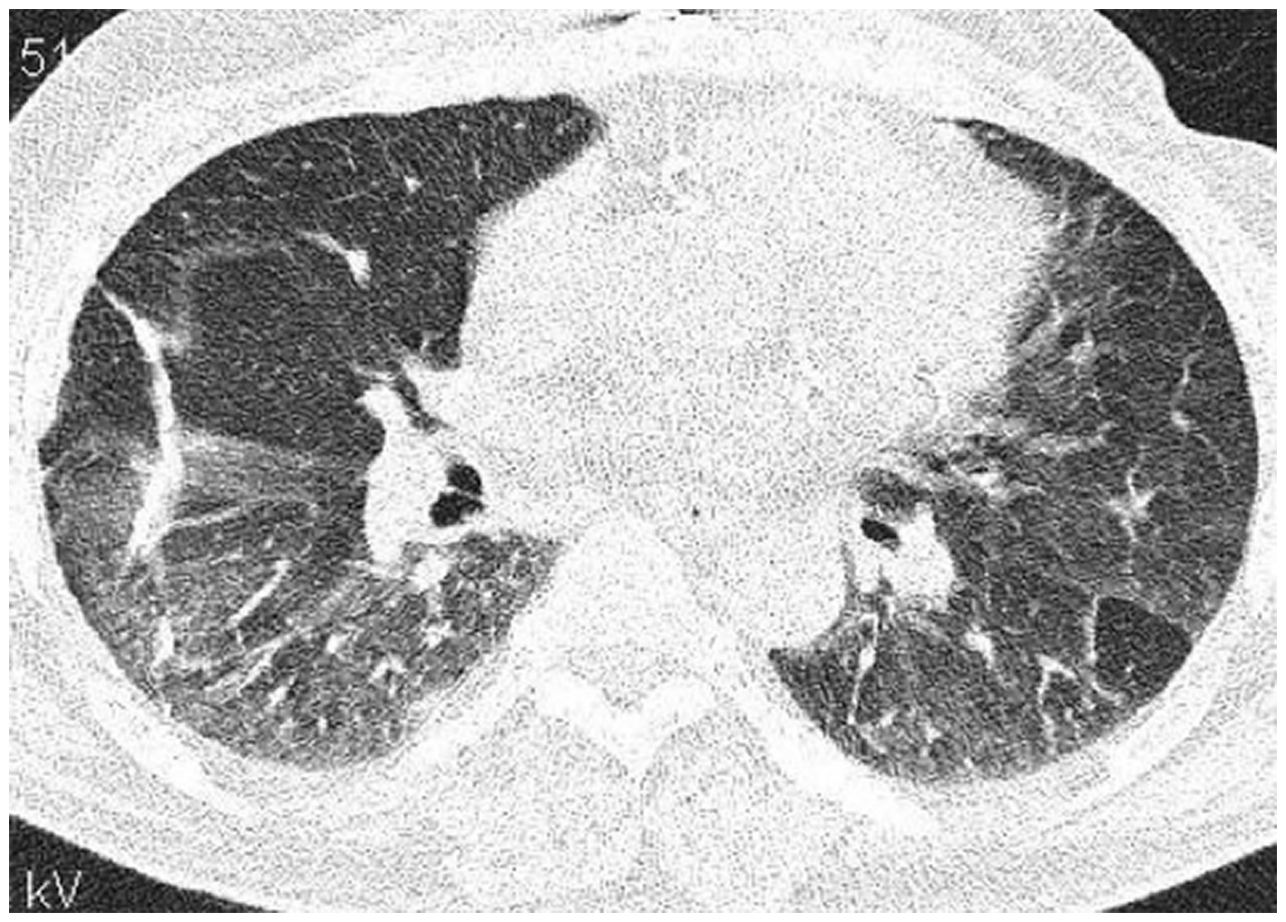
High resolution computed tomography scan of a patient with SOP in the course of dermatomyositis. In both lungs evidence of GGO with patchy distribution and parenchymal banding with “arcade” sign typical of OP. Status post lung biopsy on the right side.
